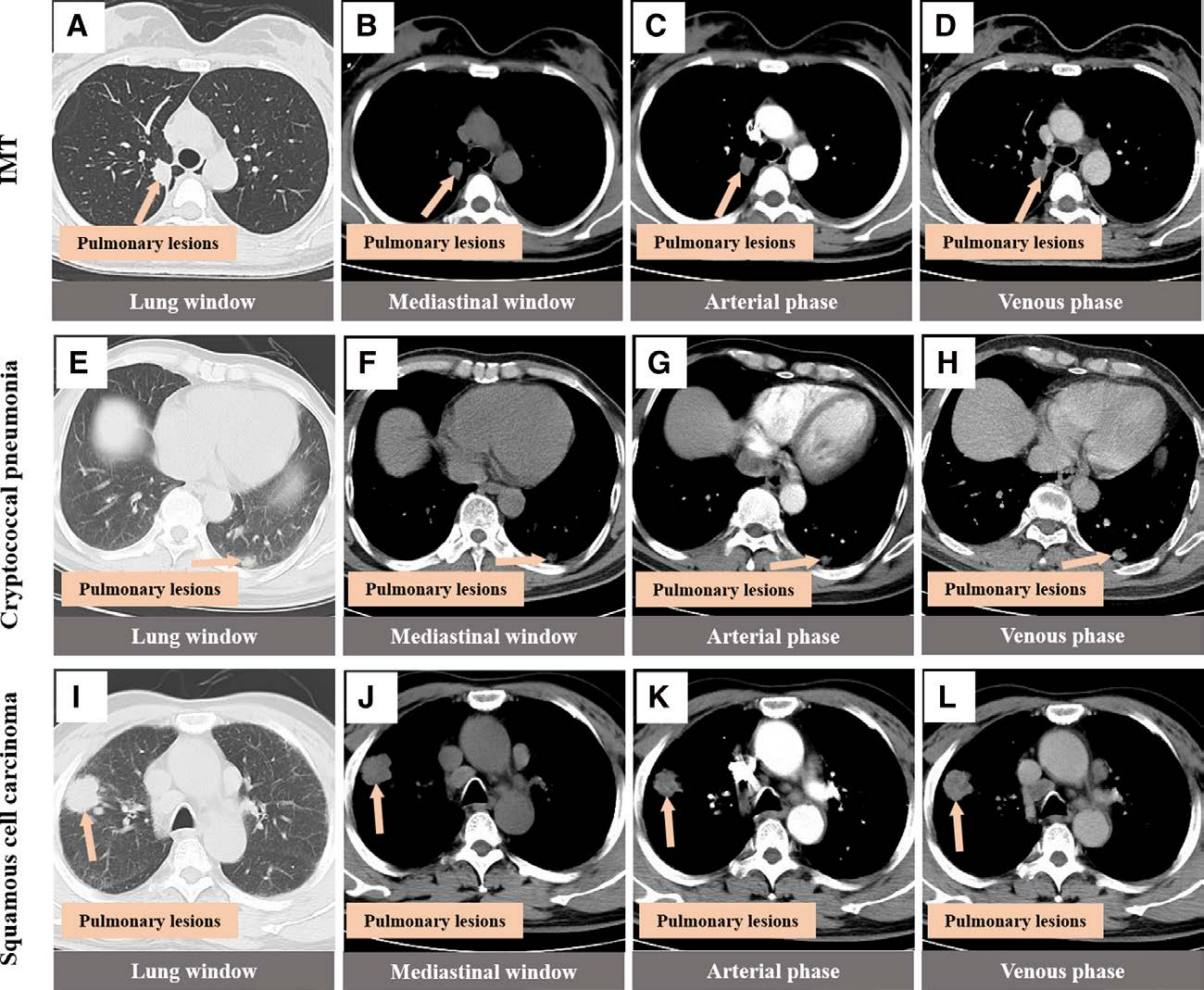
CT findings of three different lung masses note. (A–D) Oval soft tissue density nodules in the right upper lung, with shallow lobulated edges, mild to-moderate uneven delayed enhancement on enhanced scanning, and slight adjacent pleural thickening. (E–H) Oval soft tissue density nodules in the left lower lung with blurred boundary and obvious uniform enhancement on enhanced scan. (I–L) Circular soft tissue density nodules in the right upper lung with deep lobulated edges, marked uneven enhancement on contrast-enhanced scan with small areas without enhancement. CT = computed tomography.
